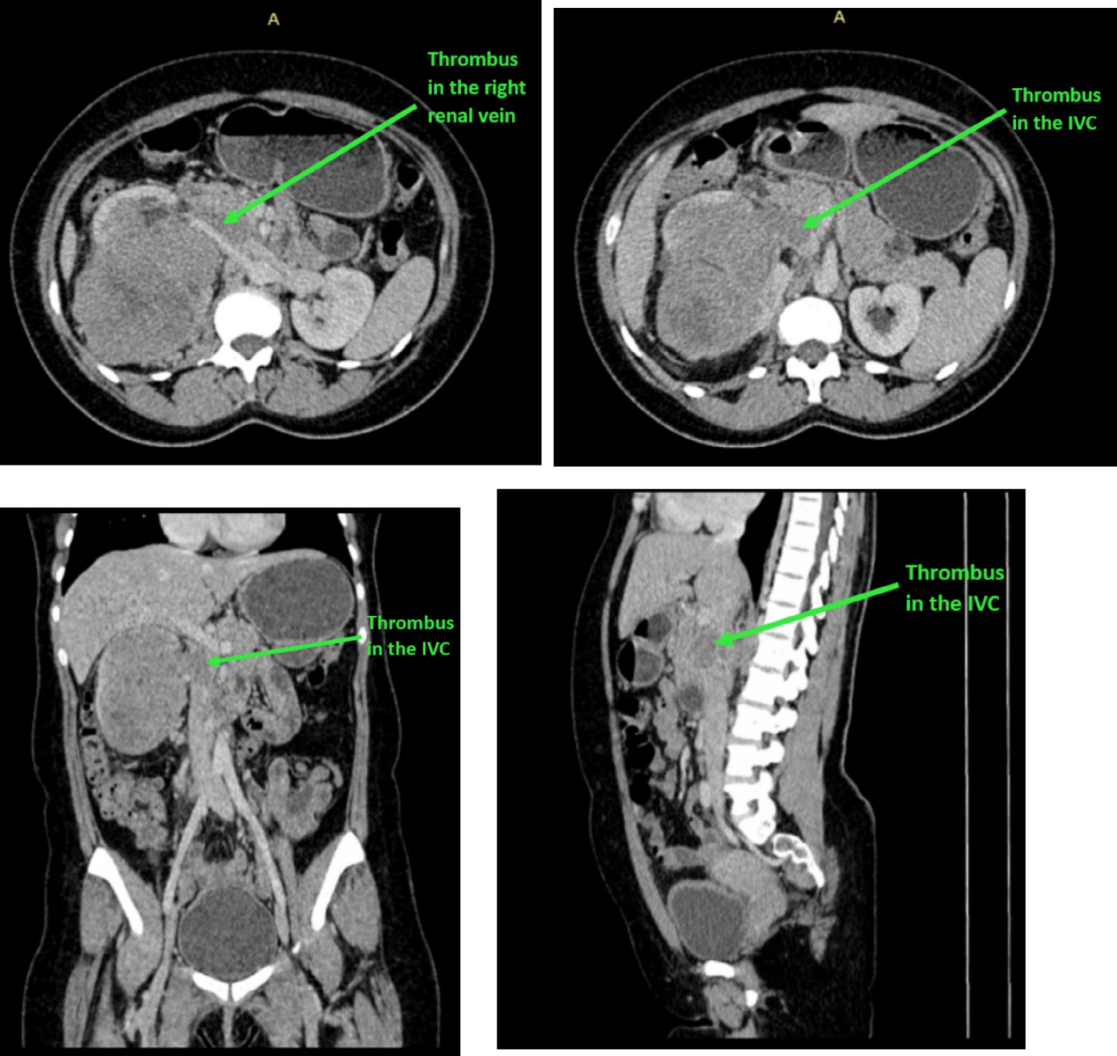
Contrasted axial, coronal, and sagittal CT scan of the abdomen showing extensive tumor thrombus filling the right renal vein and the IVC.
